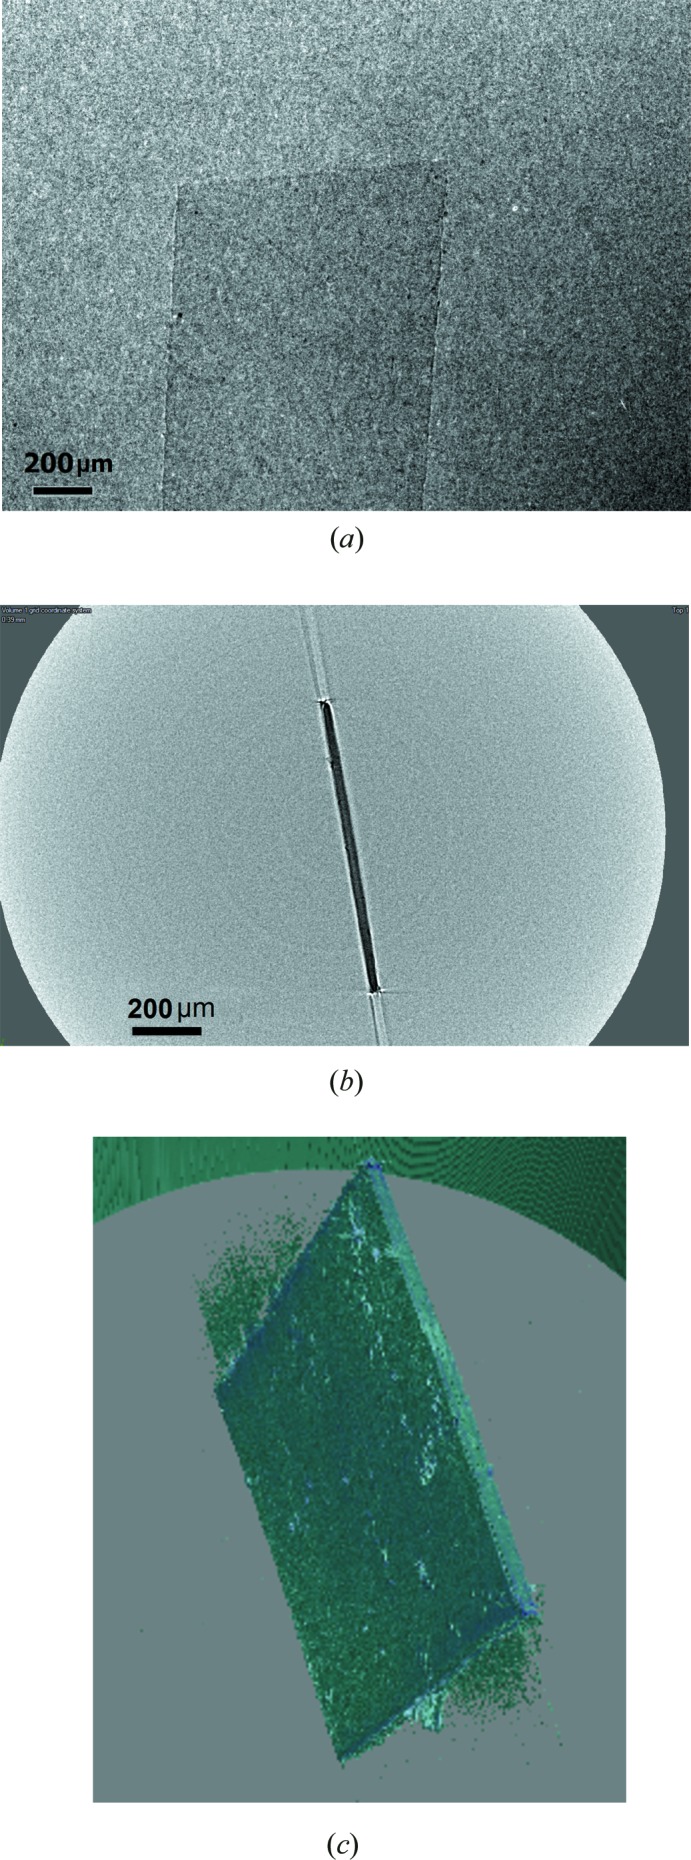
(*a*) Single projection of 30 µm-thick Kapton foil showing very poor contrast. SDD = 109.3 mm, SOD = 28 mm, effective pixel size = 1.6 µm. Total number of projections 2250. Exposure time 1.5 s, setting of X-ray tube 80 kV, 100 µA, focus size of 8 µm. (*b*) Slice from a reconstruction performed using the *Octopus* package. (*c*) Segmentation and visualization using *VGStudio* is complicated owing to ray aliasing artefacts and the remains are visible at the edges of the object.
